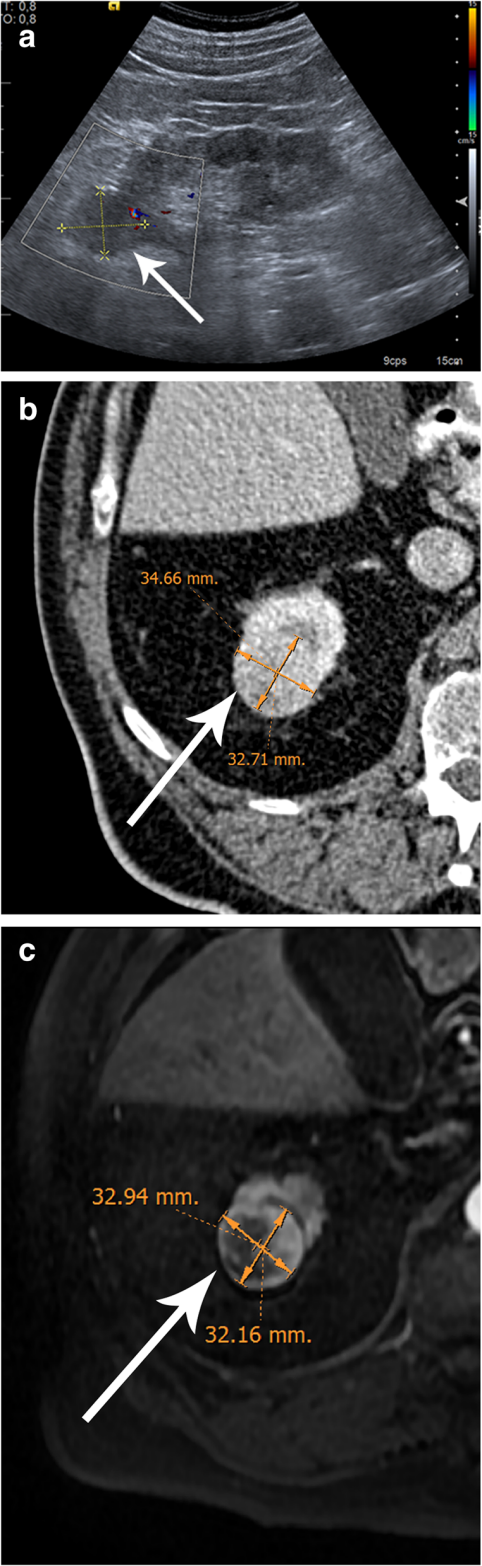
Comparison of maximum tumor diameter (MTD) in the axial plane of a small renal mass (SRM) with different imaging modalities (arrows). a Sagittal ultrasound (US) view. b Axial contrast-enhanced CT scan and (c) axial contrast-enhanced T1-weighted MR of the same renal lesion demonstrating there are no significant differences between US, CT, and MR with the use of the same plane for measuring MTD (3.33 cm, 3.46 cm, and 3.29 cm, respectively). Note that the differences between different radiological tests are less than 0.2 cm
